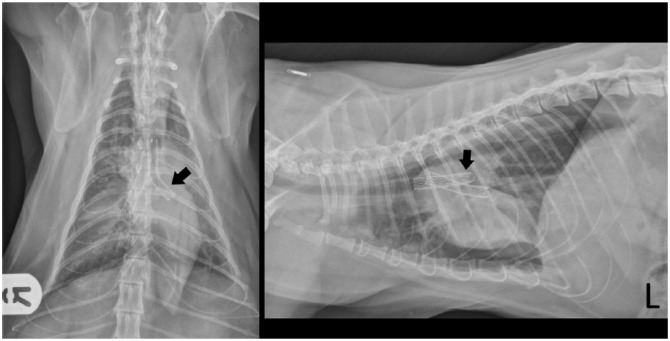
Postoperative dorsoventral and left lateral radiographic projections, obtained after needle thoracocentesis to drain a small pneumothorax. The bilateral bronchial stents are visible (left side indicated by arrow), as is some evidence of pulmonary pathology, which was considered most likely secondary to chronic bronchial obstruction. The position of the proximal portions of the stents alongside one another in the distal trachea is referred to as ‘kissing stents’ and reduces the risk of stent re-obstruction
